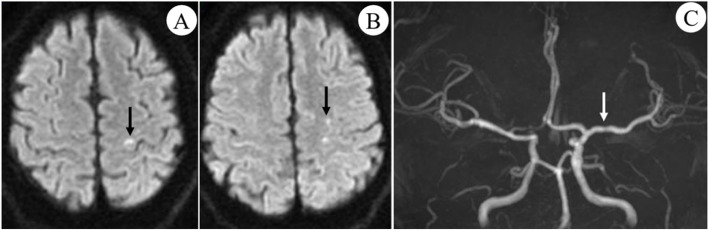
MRI-DWI and MRA findings. a, b multiple high signals on DWI located within the left prefrontal cortex and centrum semiovale (black arrows); c MRA showed mild focal stenosis on the LMCA horizontal segment (white arrow)
